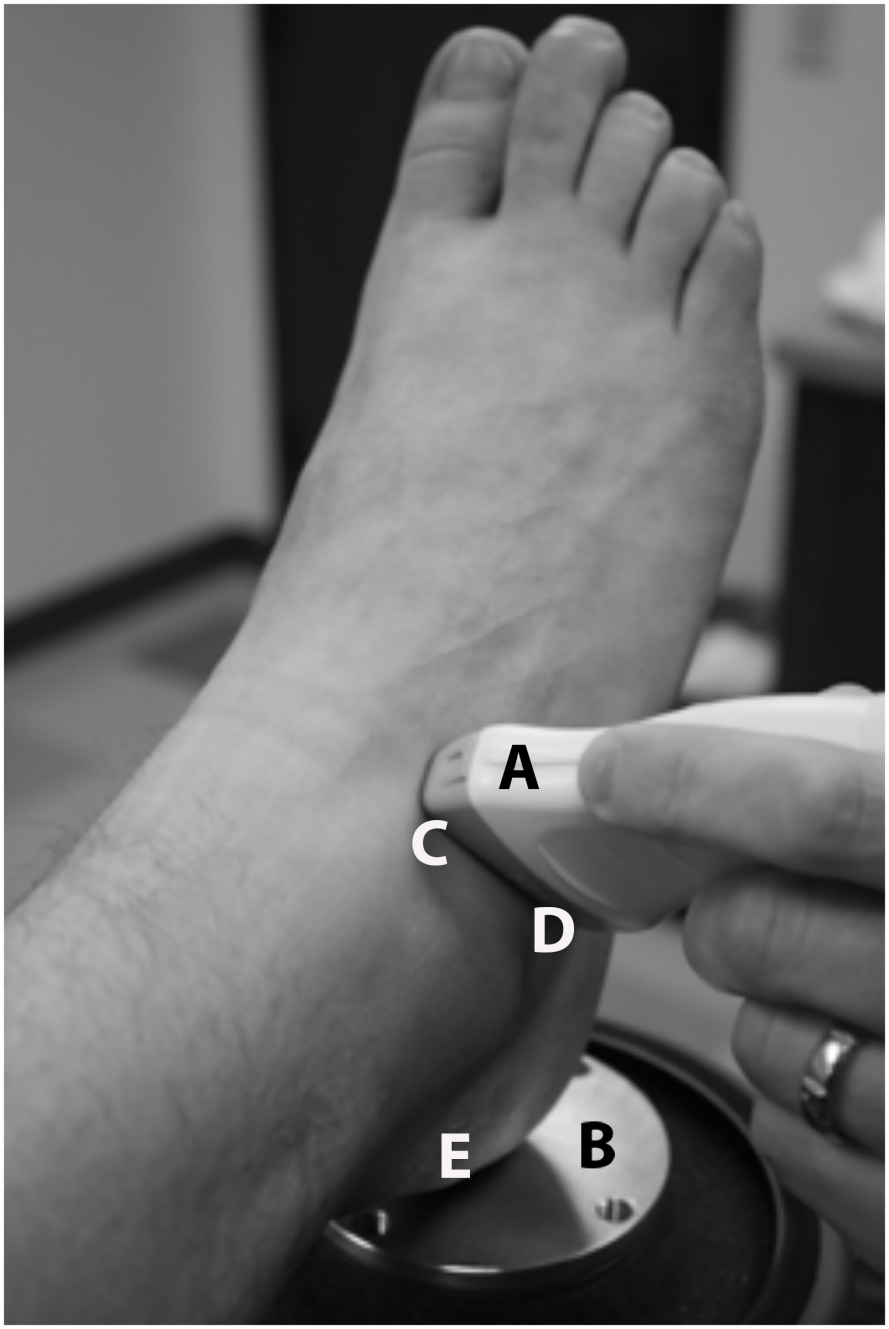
Sound head placement on the lateral STJ. “A = Ultrasound probe; B = Vibration generator platform; C = End of ultrasound probe positioned over the lateral talar neck; D = End of ultrasound probe positioned over the distal lateral calcaneus; E = Calcaneal point of contact over the vibration generator”.
